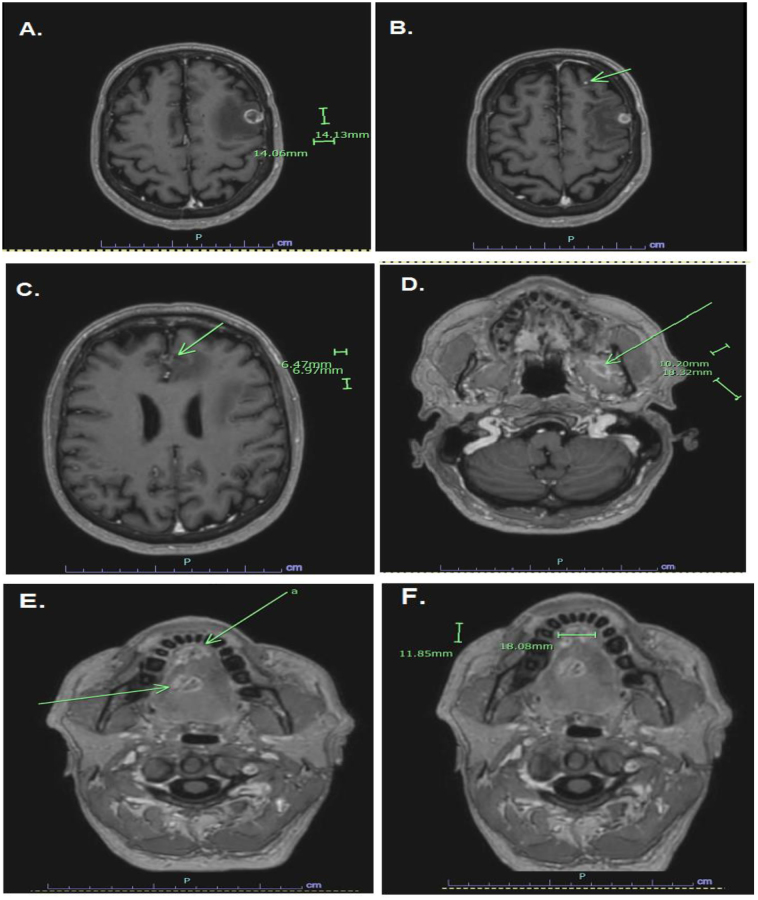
MRI of the brain. (A) 1.4 × 1.4 cm enhancing lesion in the left frontal lobe with surrounding vasogenic oedema; (B) 0.7 × 0.6 cm peripherally enhancing lesion in the left parasagittal frontal lobe with adjacent oedema and local mass effect; (C) Punctate focus of enhancement in the left parietal lobe; (D) 1.8 × 1.0 cm heterogeneously enhancing lesion in the left masticator space; (E) 1.5 × 1.8 × 1.7 cm enhancing lesion in the mid-tongue; (F) 1.2 × 1.8 cm enhancing lesion in the anterior tongue/floor of mouth.
